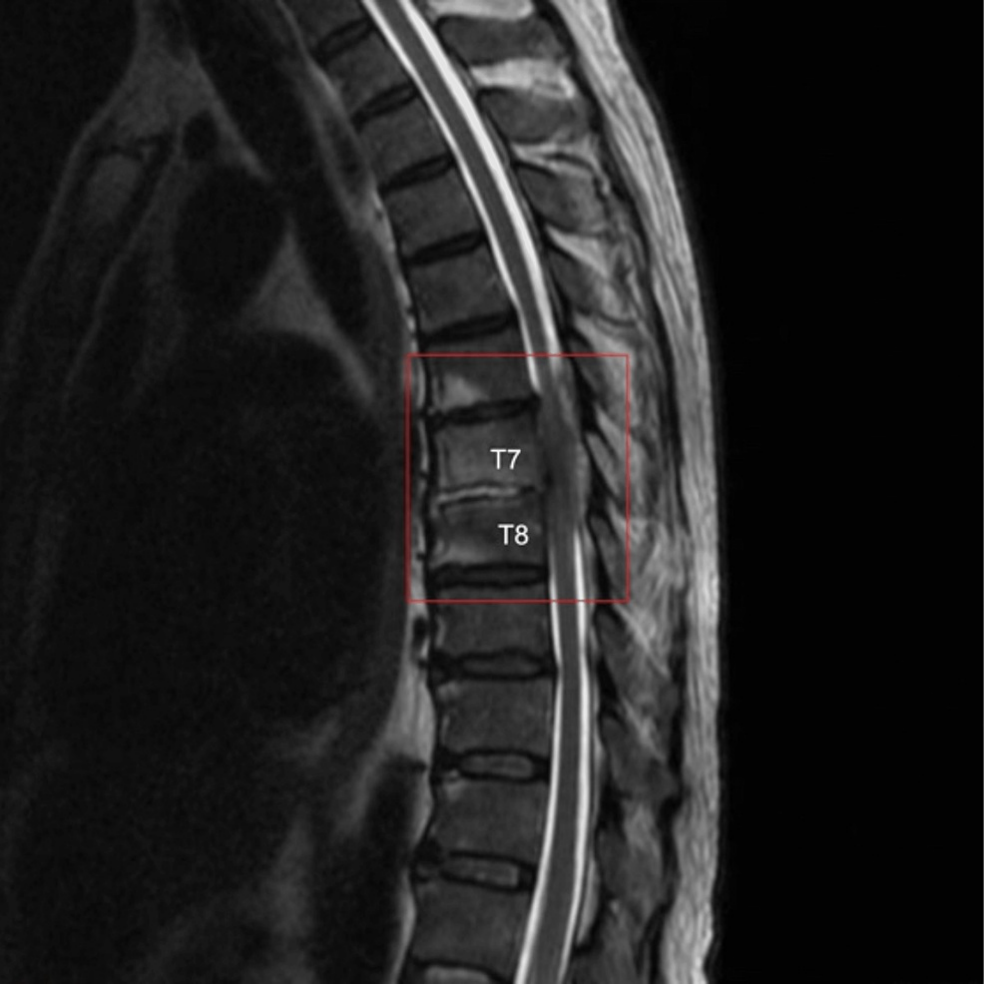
T2-weighted sagittal view revealing T7-T8 discitis/osteomyelitis with associated extensive phlegmonous enhancements and a thin dorsal epidural abscess (red box).
